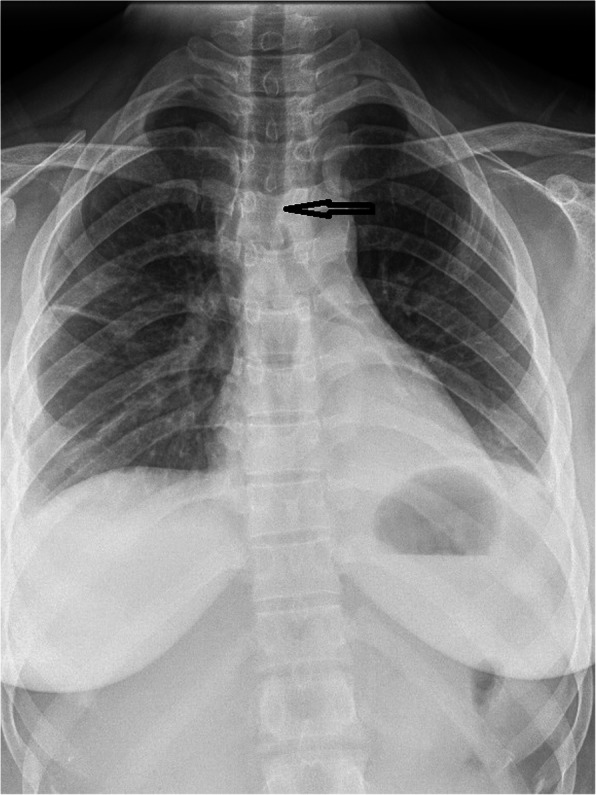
Chest X-ray showing normal post-operative change in trachea (black arrow) with no lung metastasis
